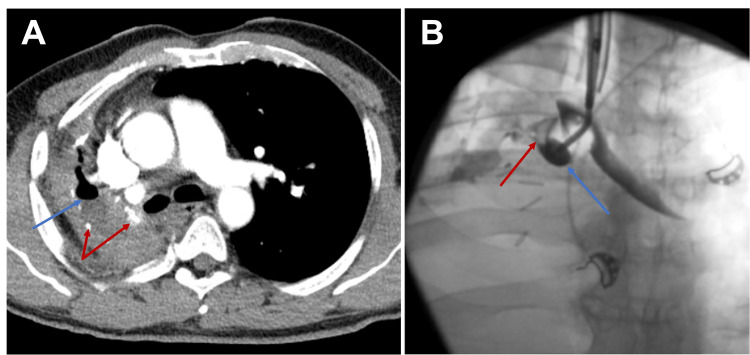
Preoperative evaluation: (A) Chest CT scan of the 59-year-old male showing calcifications (red arrows) and air-fluid (blue arrow) levels within the right post-pneumonectomy residual pleural cavity; (B) Contrast extravasation to the residual pleural space (red arrow) injected through the bronchial blocker in the right bronchial stump (blue arrow) confirming the presence of a broncho-pleural fistula.
